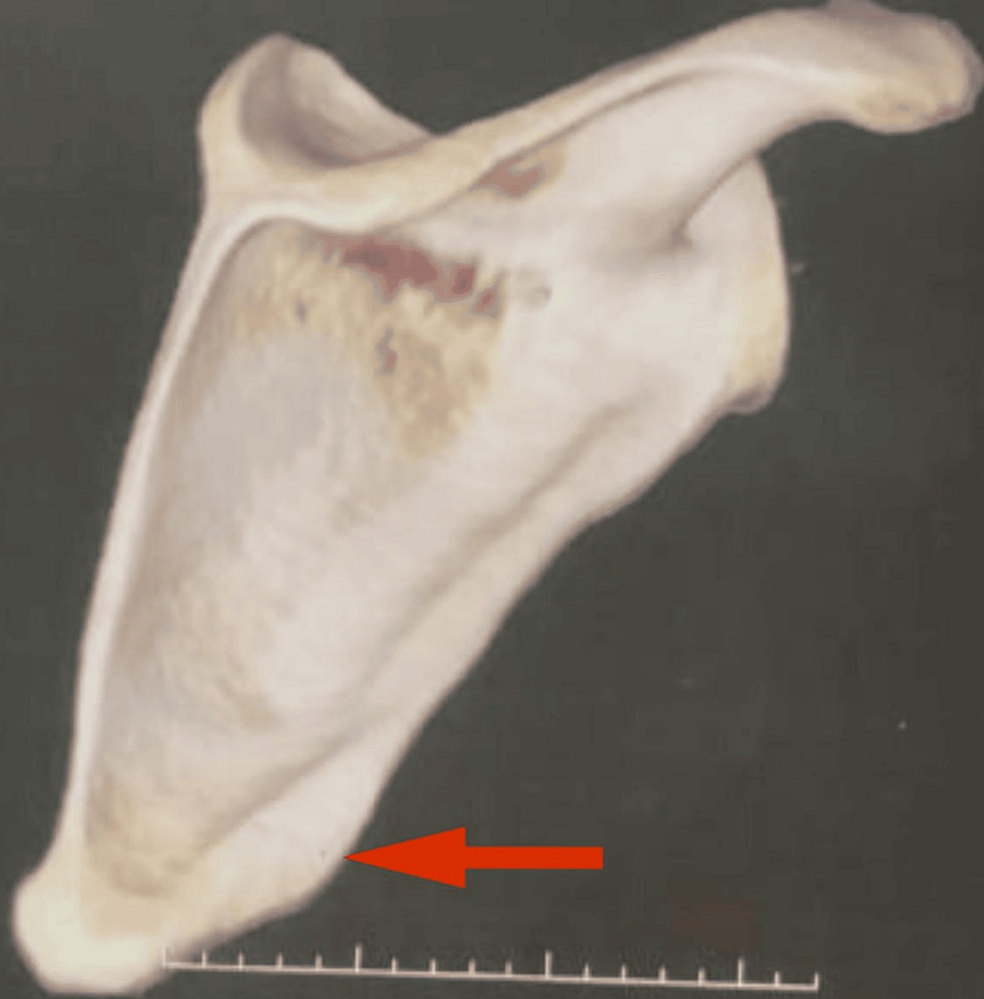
MRI of the right shoulder revealed an inferomedial border exophytic bony outgrowth measuring 1.7 cm in length and 6 mm in thickness on the right side of the scapula (red arrow)
